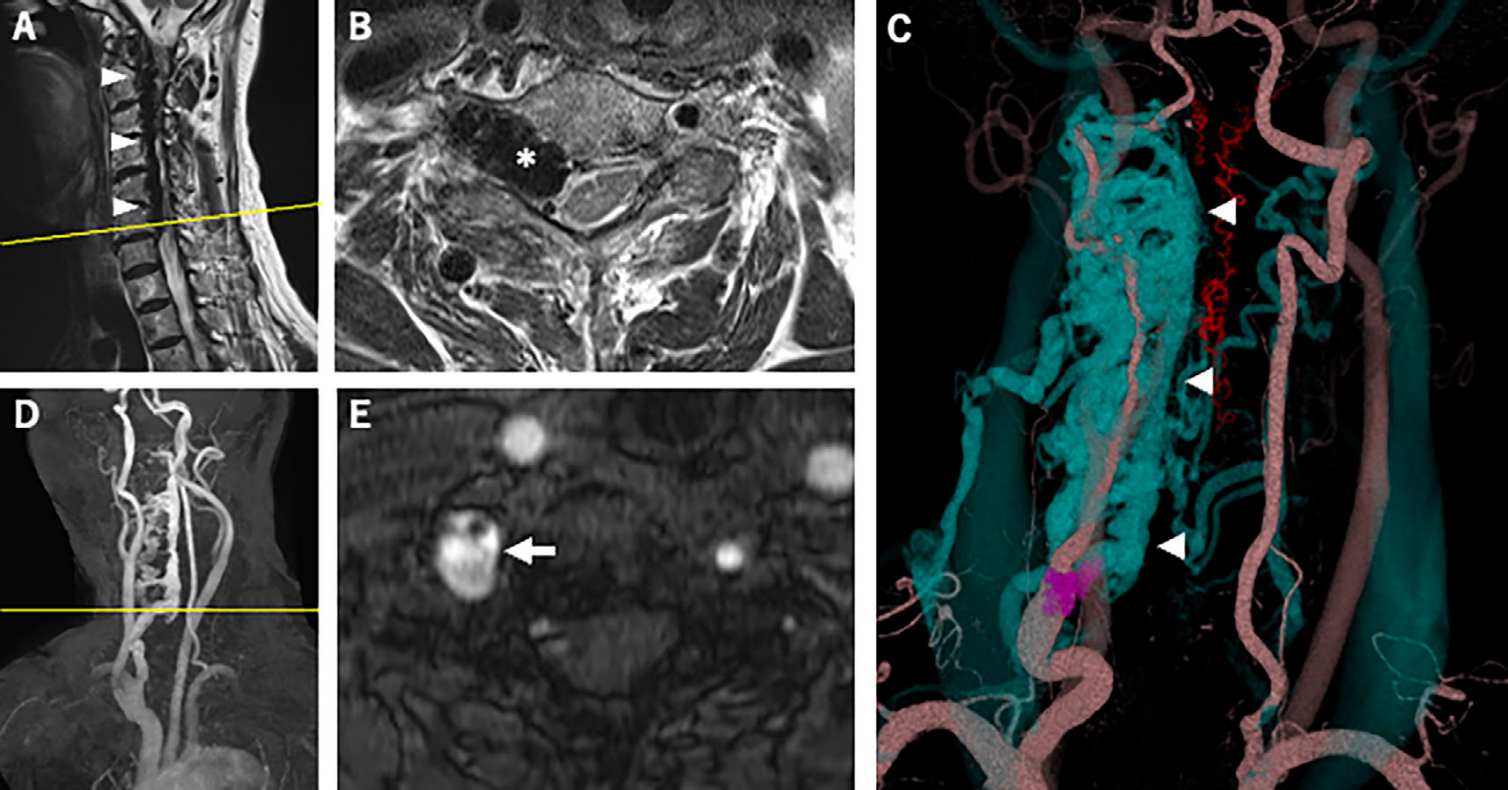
Magnetic resonance imaging and computed tomography angiography. (A) Sagittal T2-weighted imaging of the cervical spine showed the dilated internal vertebral venous plexus in the right side of the spinal canal from the C1 to C6/7 levels (arrowheads). (B) (the cross-section image of A at the yellow line) Axial T2-weighted imaging of the cervical spine showed the dilated right intervertebral vein (asterisk) at the C6/7 level compressing the spinal cord, and high signal intensity around the right AH at the same level. (C) Three-dimensional reconstruction of contrast-enhanced computed tomography revealed a dilated intervertebral vein and anterior internal vertebral venous plexus (blue, arrowheads). Arteriovenous fistula at the C7 level (purple) was also shown. (D) Oblique view of maximum-intensity projection cervical MRA showed a dilated right intervertebral vein and anterior internal vertebral venous plexus. The yellow line indicates the cross-sectional position of (E). (E) Axial image of time-of-flight cervical MRA showed an arteriovenous fistula at the C7 level (arrow). AH, anterior horn; MRA, magnetic resonance angiography.
